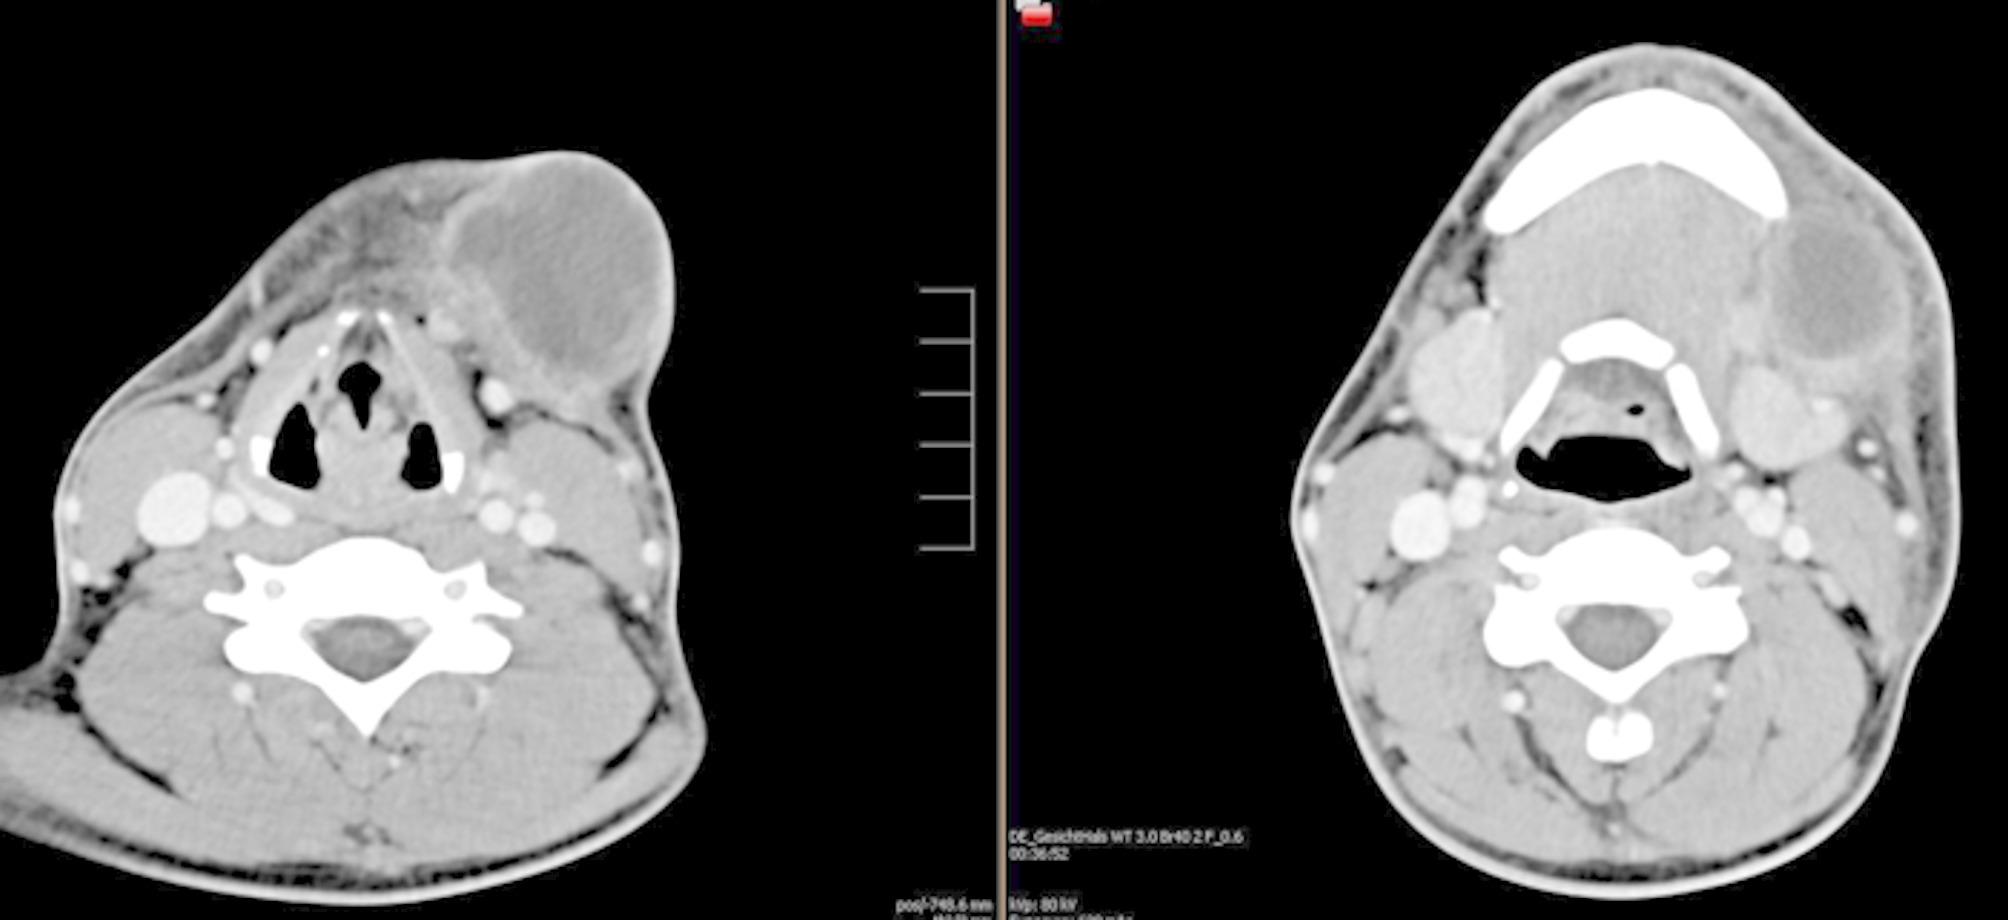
Axial contrast-enhanced computed tomography (CT) scan demonstrating a perimandibular abscess on the left side. The image shows a hypodense, rim-enhancing fluid collection adjacent to the left mandibular body, with surrounding soft tissue swelling and signs of inflammatory infiltration
